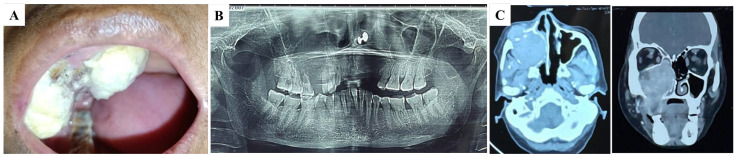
Clinical, radiographical, and CT scans of the oral and maxillofacial region. (A) Intra-oral view of the initial lesion. A double lobe nodule is observed in the right maxilla. (B) A panoramic radiograph displays a radiolucent lesion with an indistinct margin, suggesting osteolysis between teeth 12 and 17. (C) A CT scan of the maxillofacial area shows a soft tissue lesion in the right maxillary sinus with heterogeneous enhancement and destruction of the bony wall.
